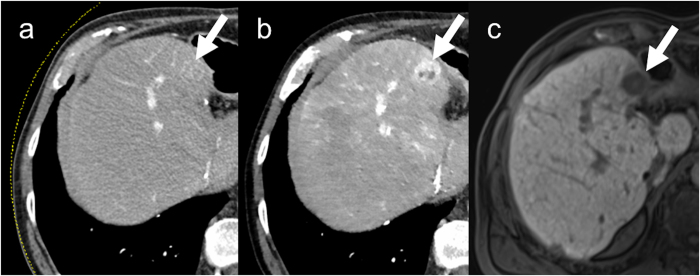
HCC detected on MRI in hepatobiliary phase (c). The arrow shows the HCC. (a) Barely visible at a tube voltage of 120 kV. (b) Effectively visualized at 80 kV with iterative image reconstruction.
